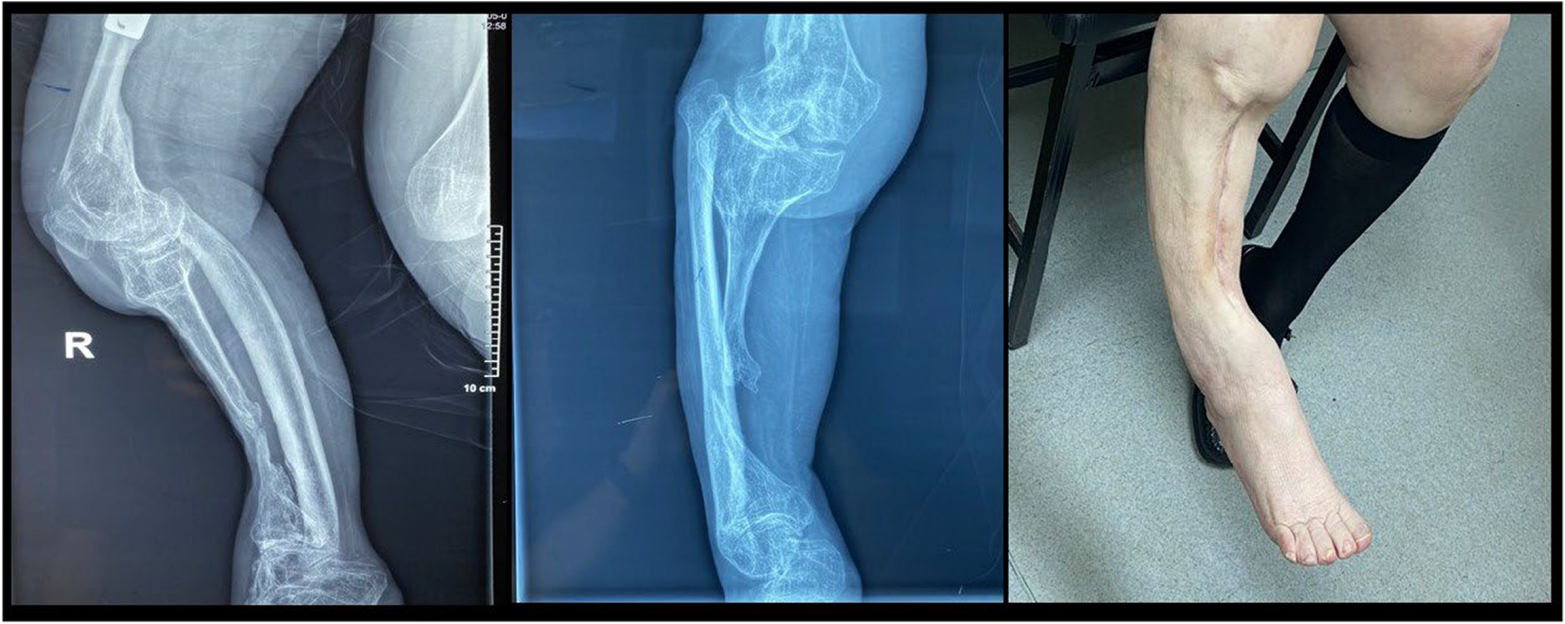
Radiographic imaging and clinical presentation.
